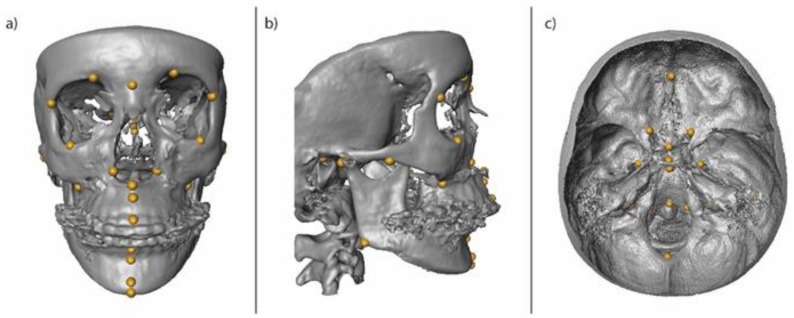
Volume rendering of a 3D skull in a frontal (a), lateral (b), and axial section (c) view of the cranial base, including the anatomical landmarks that were annotated on the CBCT images and used for the morphometric analysis. Each landmark is indicated by a yellow dot [55]. The list of landmarks can be found in Supplementary Table S2.
